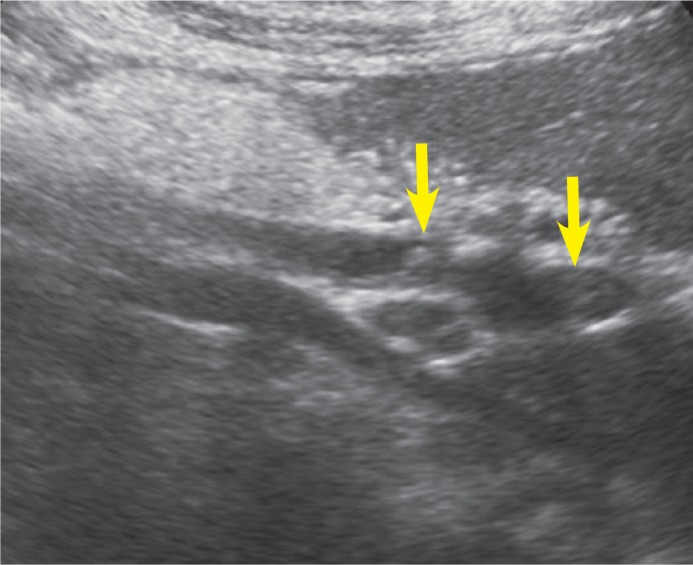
Sonogram of the common bile duct depicts curvilinear echogenic parasites *(arrow)*.
